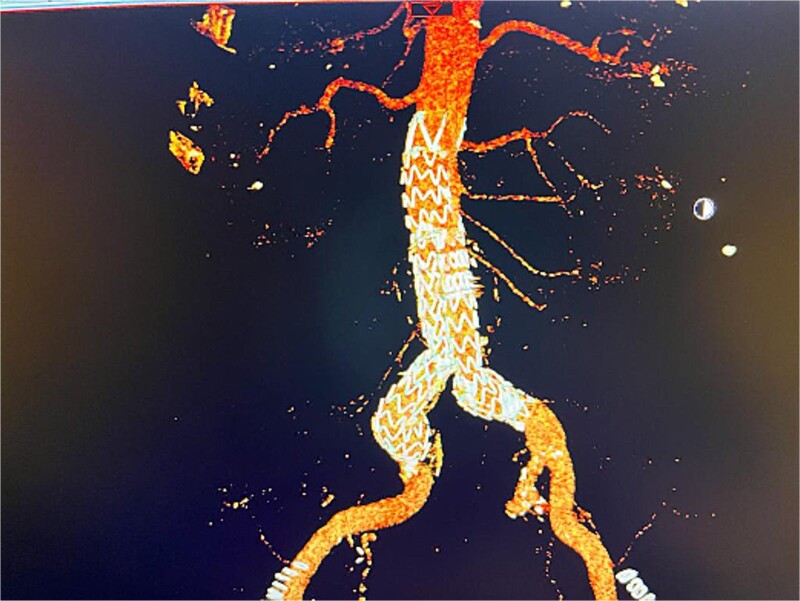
Postoperative 3D reconstruction CTA image of the EVAR without any sign of endo leak.
